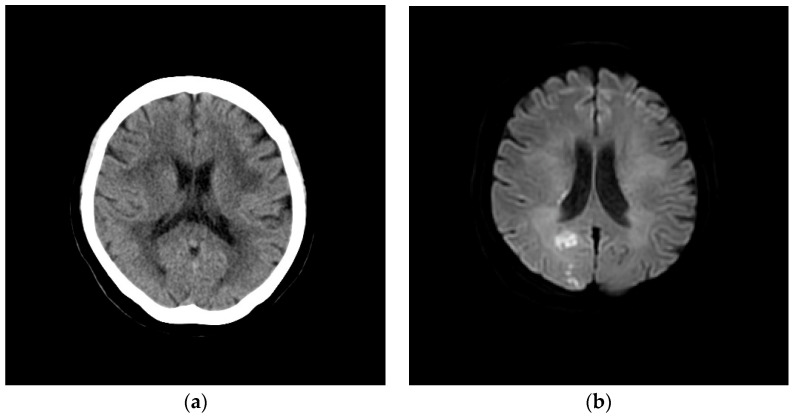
The brain computed tomography (a) and magnetic resonance imaging with diffusion-weighted imaging (b) results of the patient, a 69-year-old woman.
